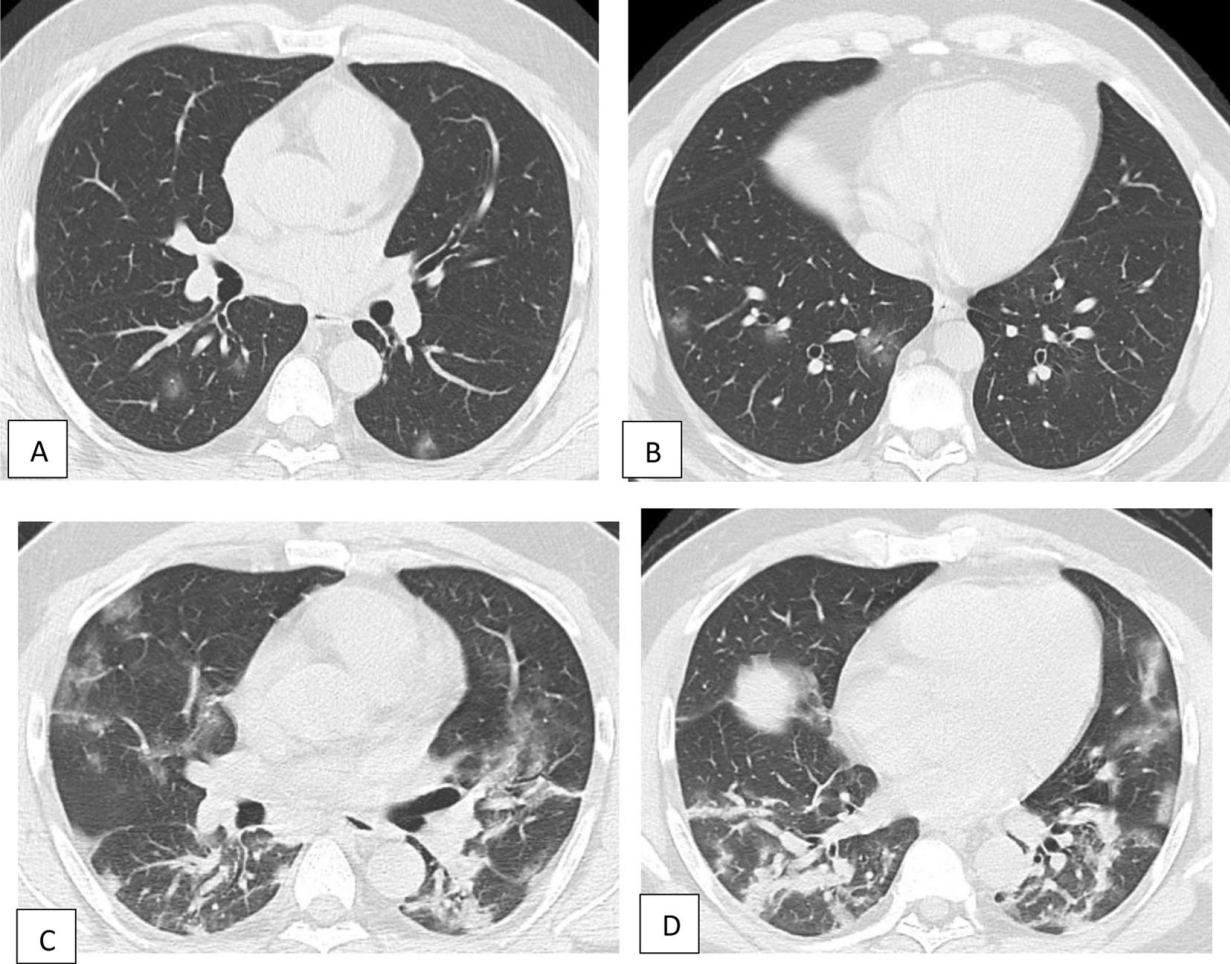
Unenhanced chest CT of a 42-year-old male patient with COVID-19. a, b Axial lung window images showing subpleural and broncho-vascular small areas of GGO with CT score 5. c, d Axial lung window images after 5 days showing the progression of the disease with increase in the number and size of the GGO areas with the development of frank areas of consolidation and fibrosis with CT score of 17
